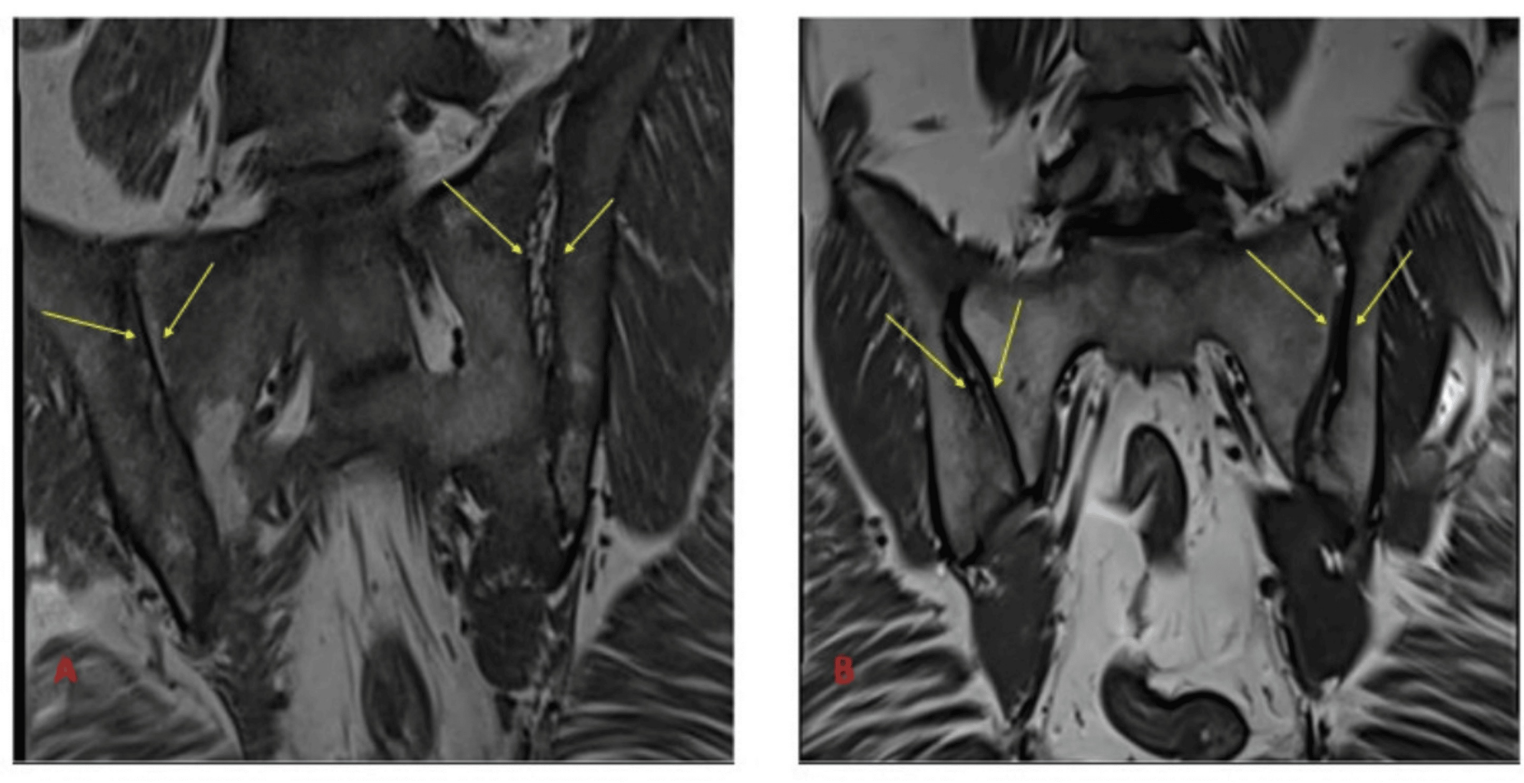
MRI T1-weighted image of sacroiliac joints before and after treatment (A) The baseline T1-weighted image highlights significant sacroiliitis with evident joint inflammation, effusion, and structural abnormalities. These findings are consistent with active disease and inflammatory damage prior to treatment initiation. (B) The follow-up T1-weighted image taken after 12 months of infliximab therapy demonstrates a marked resolution of inflammation and significant improvement in joint integrity, with no new erosive changes observed. MRI: magnetic resonance imaging
